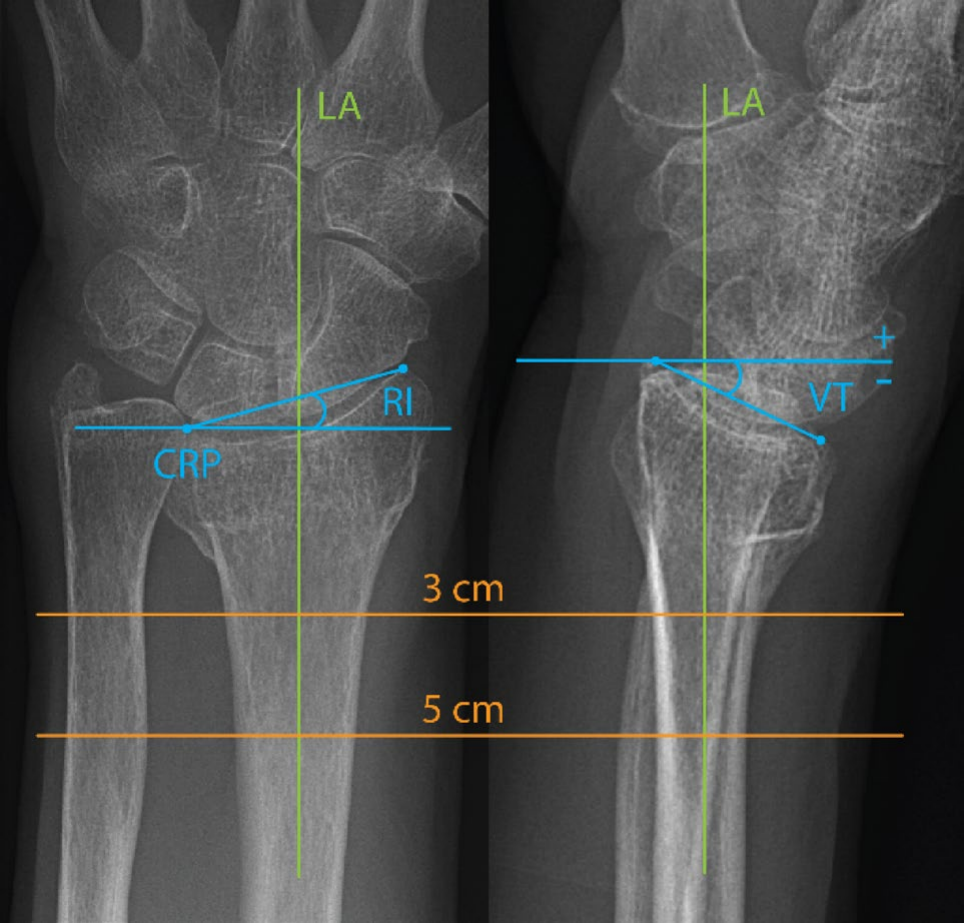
Measurement of radial inclination (RI) and volar tilt (VT) on standard radiographs. The longitudinal axis of the radius (LA) is defined as the central line through the radial shaft between 3 and 5 cm proximal to the central reference point (CRP). The CRP is located midway between the volar and dorsal ulnar corners of the articular surface. Radial inclination is measured on the postero-anterior view as the angle between the line drawn from the CRP to the radial styloid and a line perpendicular to the LA. Volar tilt is measured on the lateral view as the angle between the line connecting the volar and dorsal rims of the articular surface and a line perpendicular to the LA. A negative volar tilt value indicates a dorsal angulation.
